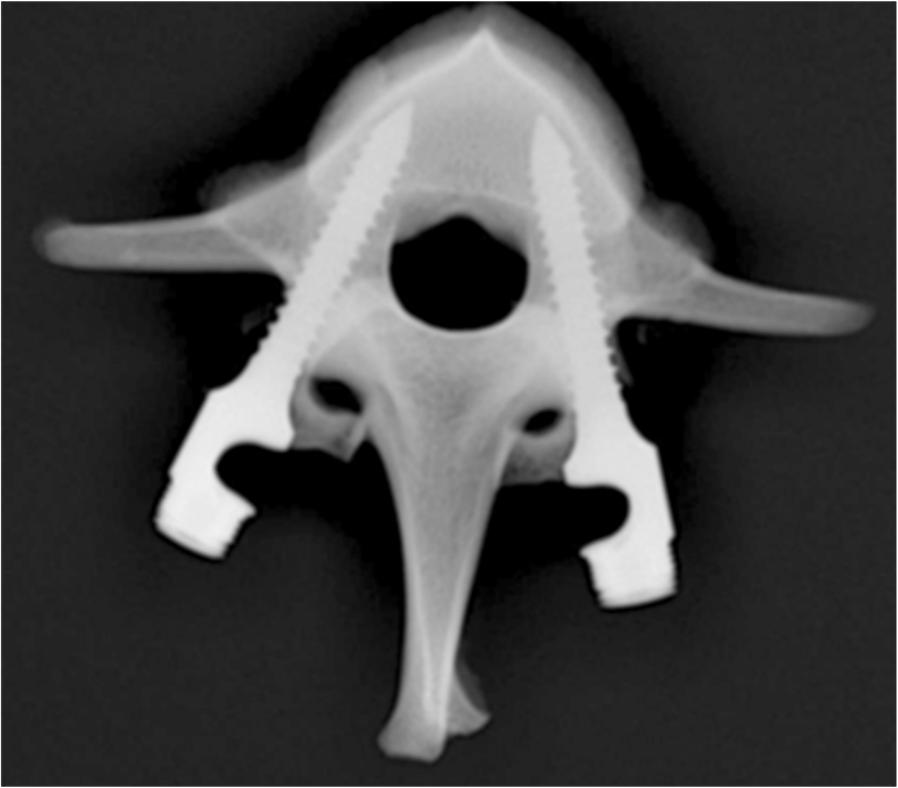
Radiograph of the lumbar vertebra with pedicle screws implanted bilaterally
